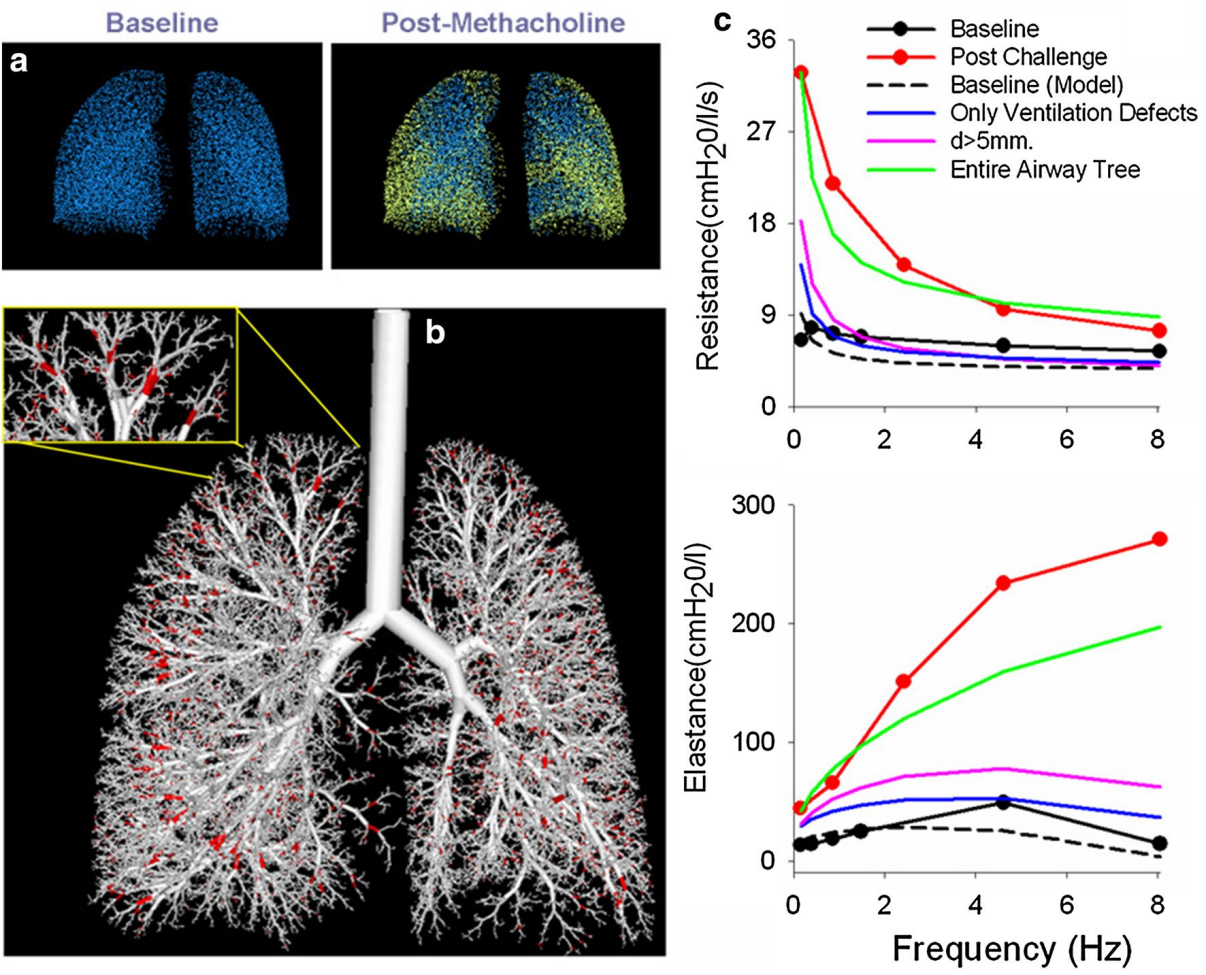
Overview of image functional modeling. a Terminal units are defined from the airway tree model which are subsequently mapped to the ventilation defects obtained from HP ^3^He MRI with ventilated terminal units depicted in *blue* and the nonventilated terminal units depicted in *yellow*. b Through a recursive algorithm, the largest airway is determined and designated for closure depicted in *red*. c Constriction patterns are applied to the airway tree model to best match measured R_L_ and E_L_ (reproduced with permission from [15])
